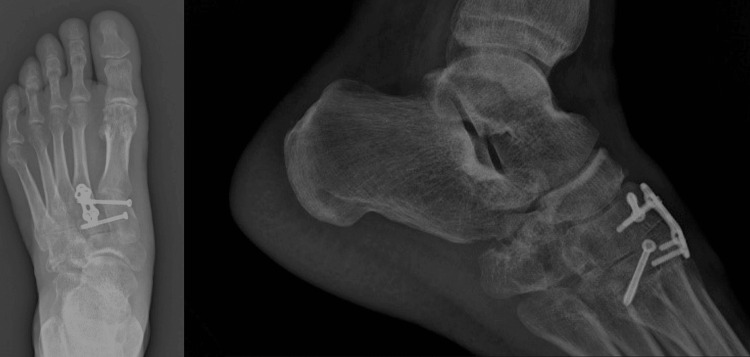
Foot radiographs three months postoperatively
